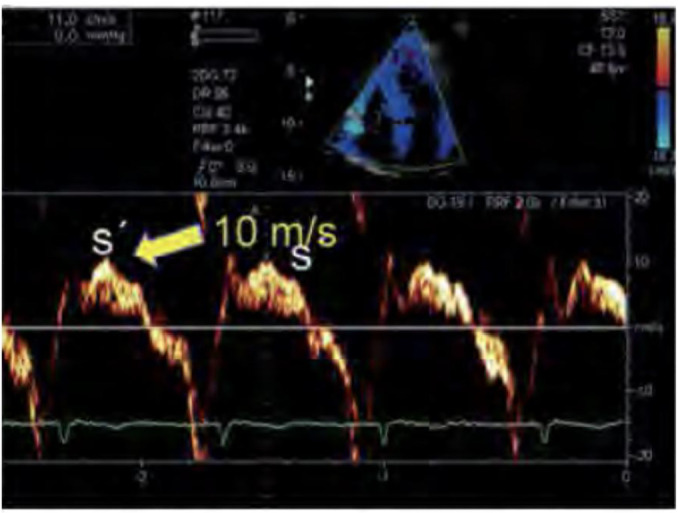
Tissue Doppler imaging of the peak velocity of tricuspid annulus
